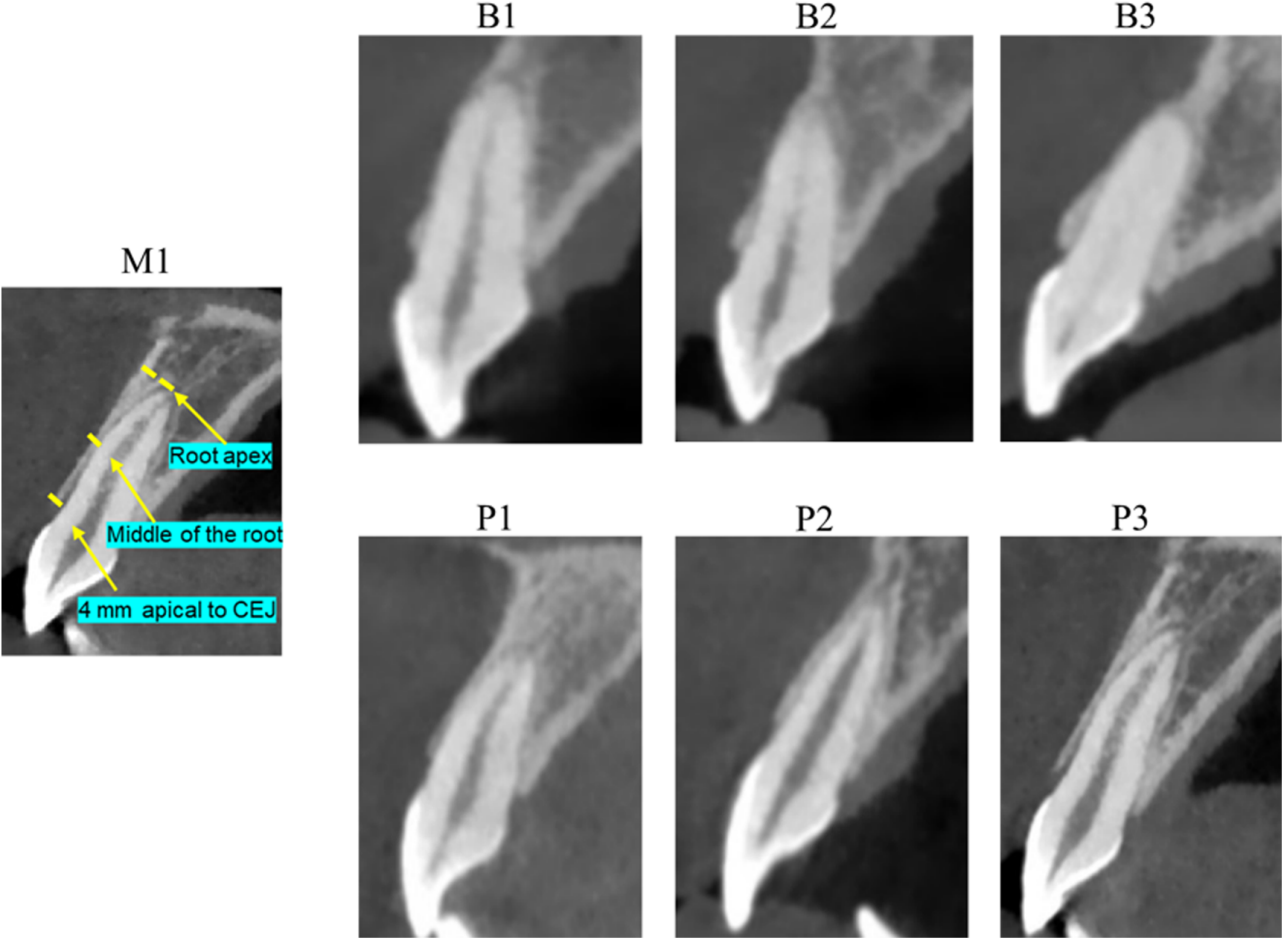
CBCT measurement images of the BBW of maxillary central incisors and CBCT images of various anatomical types of the BBW.
